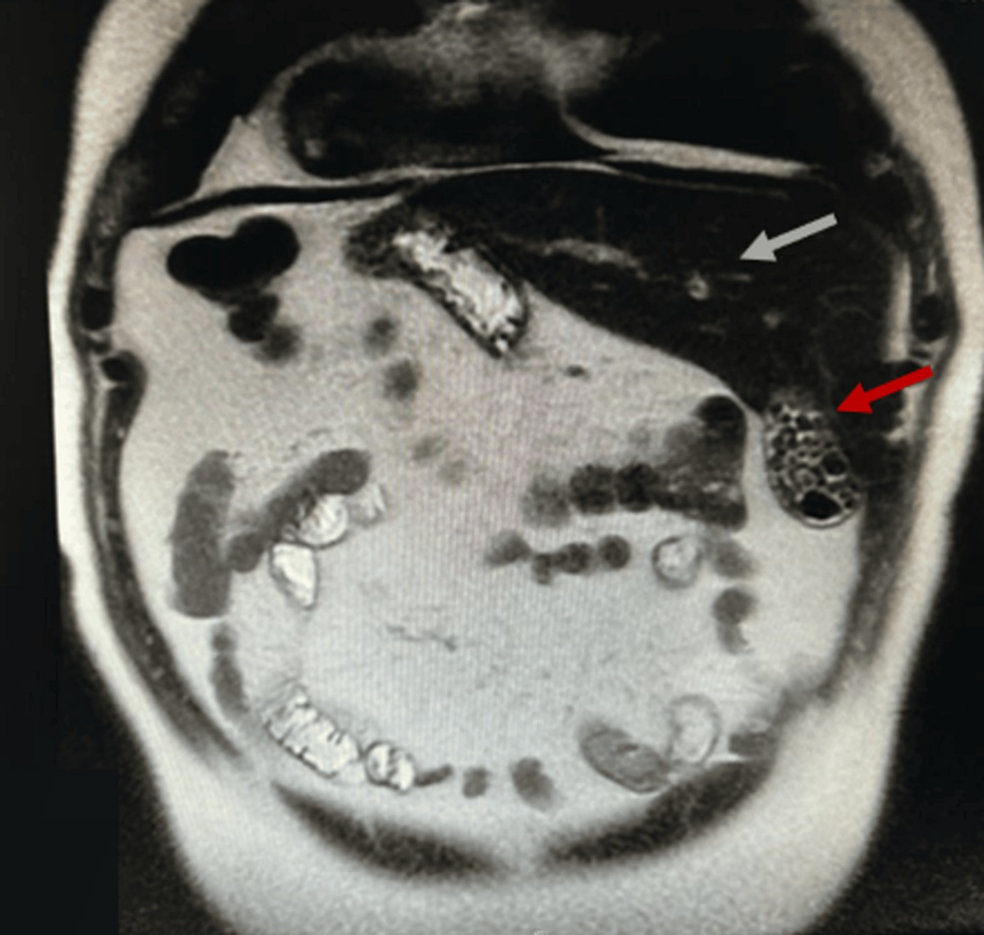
MRI, T2 weighted, coronal plane Grey arrow: Liver in the sinistroposition Red arrow: Subhepatic gallbladder, on the left side of the abdomen
